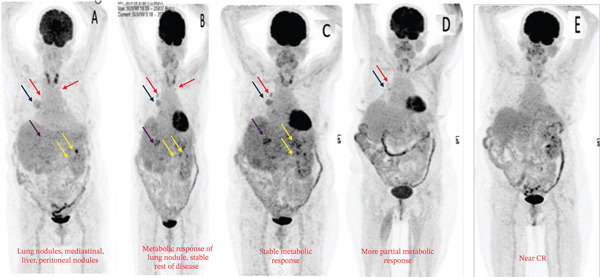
PET‐CT scans in (A) July 2023, (B) October 2023, (C) February 2024, (D) July 2024, and (E) April 10, 2025 (blue arrows: mediastinal nodes; orange arrows: pulmonary nodules; red arrows: liver nodules; and yellow arrows: peritoneal nodes).
